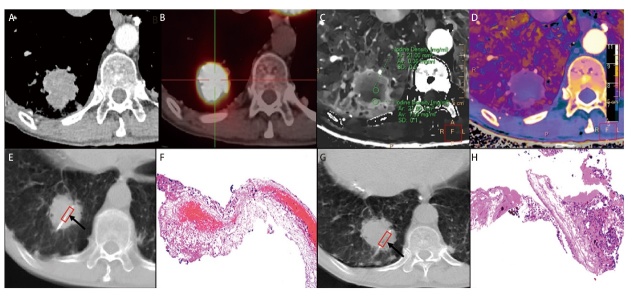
常规增强CT图像、PET/CT图像、能量CT参数图像指导肺癌穿刺活检。A-D：分别为常规动脉期CT图像、PET/CT图像、动脉期碘密度图像、动脉期CT_40 keV_/有效原子序数图融合图像。右肺下叶肿瘤常规增强CT上呈均匀密度，PET/CT上呈均匀高代谢。碘密度图显示肿瘤边缘碘密度为1.09 mg/mL，肿瘤中心碘密度为0.36 mg/mL。在CT_40 keV_图与有效原子序数图融合图像上，肿瘤内伪彩明显不均匀，肿瘤边缘颜色较肿瘤中心颜色亮。E：显示穿刺针槽（箭所指红框内）位于肿瘤中心。F：与E相对应的活检标本镜下放大图像（HE染色，×100），显示出血性坏死多且肿瘤细胞少，不足以明确诊断。G：显示穿刺针槽（箭所指红框内）位于肿瘤边缘。H：与G对应的活检标本镜下放大图像（HE染色，×100），显示有足够的肿瘤细胞可供明确诊断。由此可见，比起常规增强CT及PET/CT图像，能量CT碘密度图、CT_40 keV_图与有效原子序数图融合图像能够提供肺癌瘤内更清晰的细节，可精确指导肺癌穿刺活检。注：CT_40 keV_图为虚拟单能级40 keV图。
